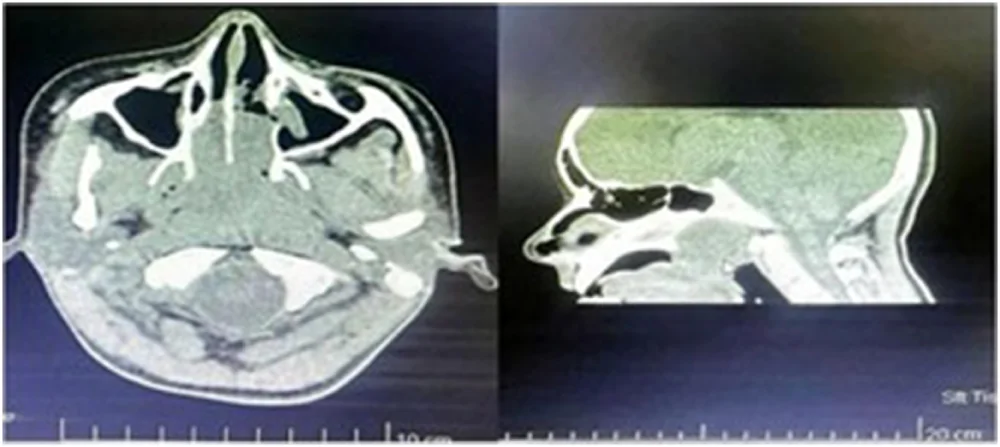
Head CT scan: Left axial CT shows a 3.6 × 3.3 × 3.0 cm nasopharyngeal mass obliterating the nasopharyngeal air columns. Mucosal thickening is noted in the maxillary and ethmoidal sinuses, with air foci in nasal cavities. Right sagittal CT reveals the mass displacing the soft palate inferiorly, causing airway obstruction. Paranasal sinus mucosal thickening is also seen.
